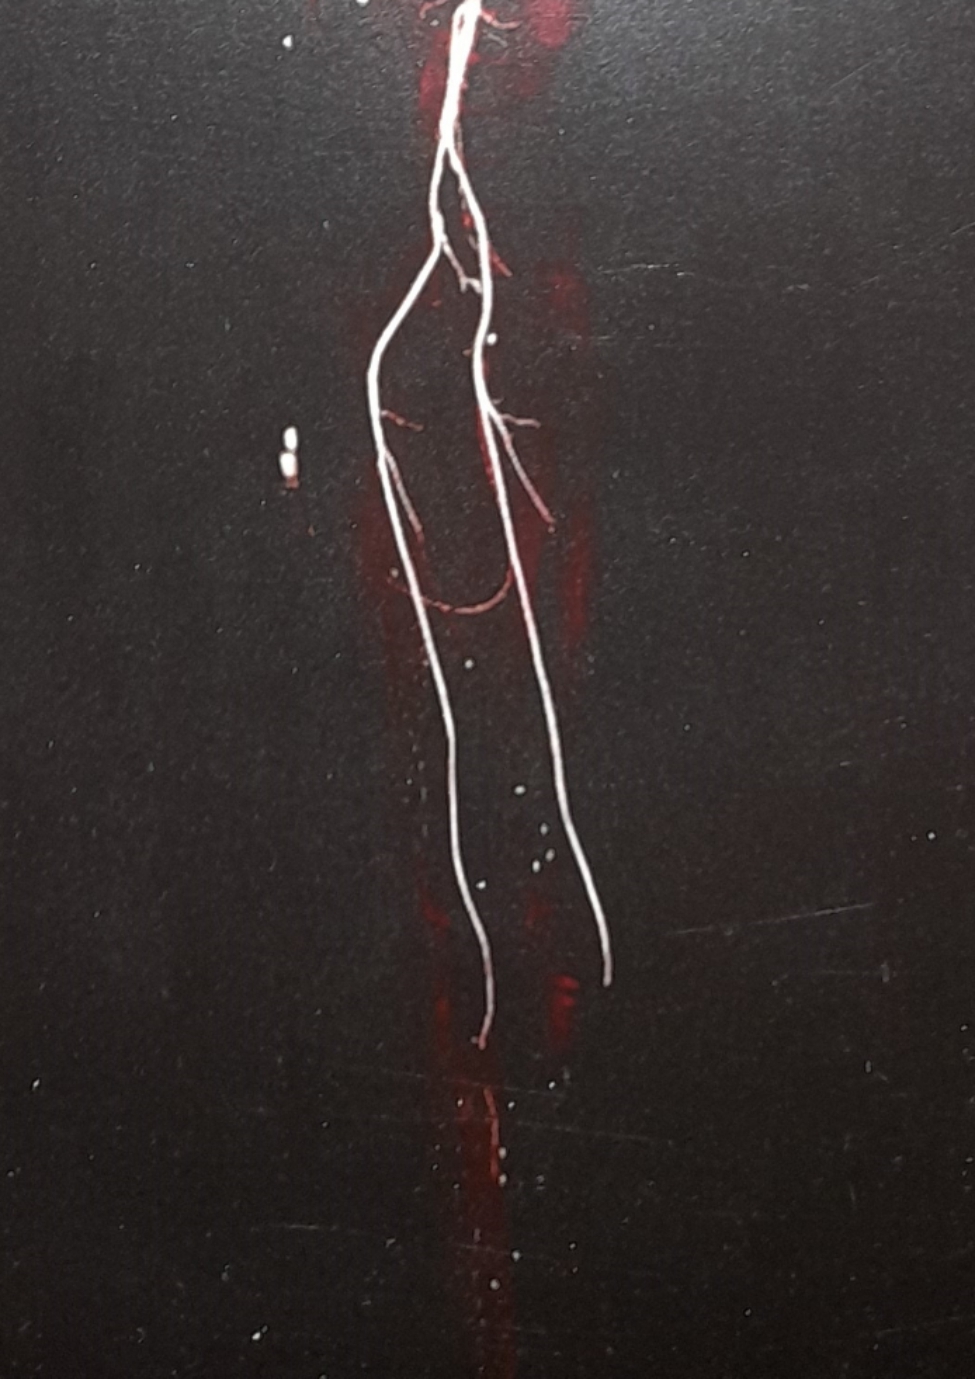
CT angiography of lower limbs showing obliteration of infrapopliteal arteries (the anterior tibial, posterior tibial and peroneal)
